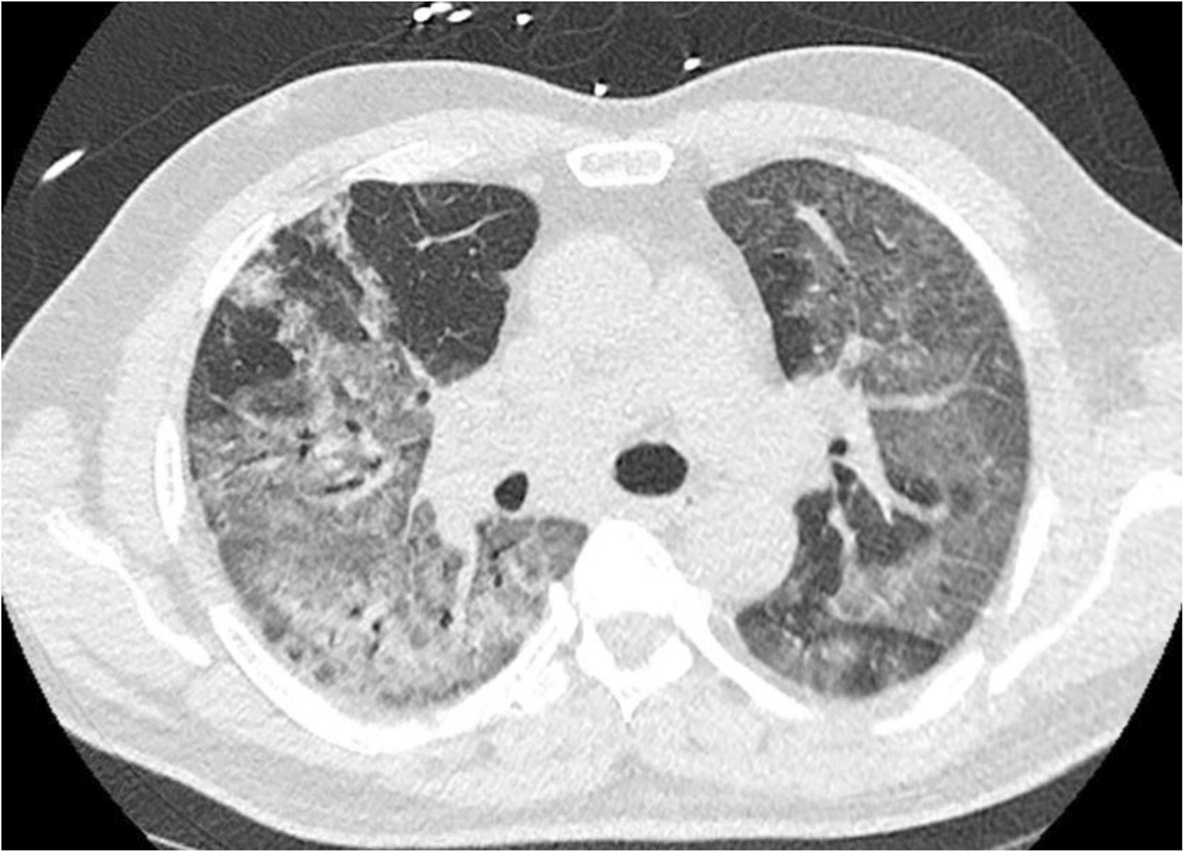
Chest computed tomography showing diffuse bilateral ground-glass opacities with limited consolidations
